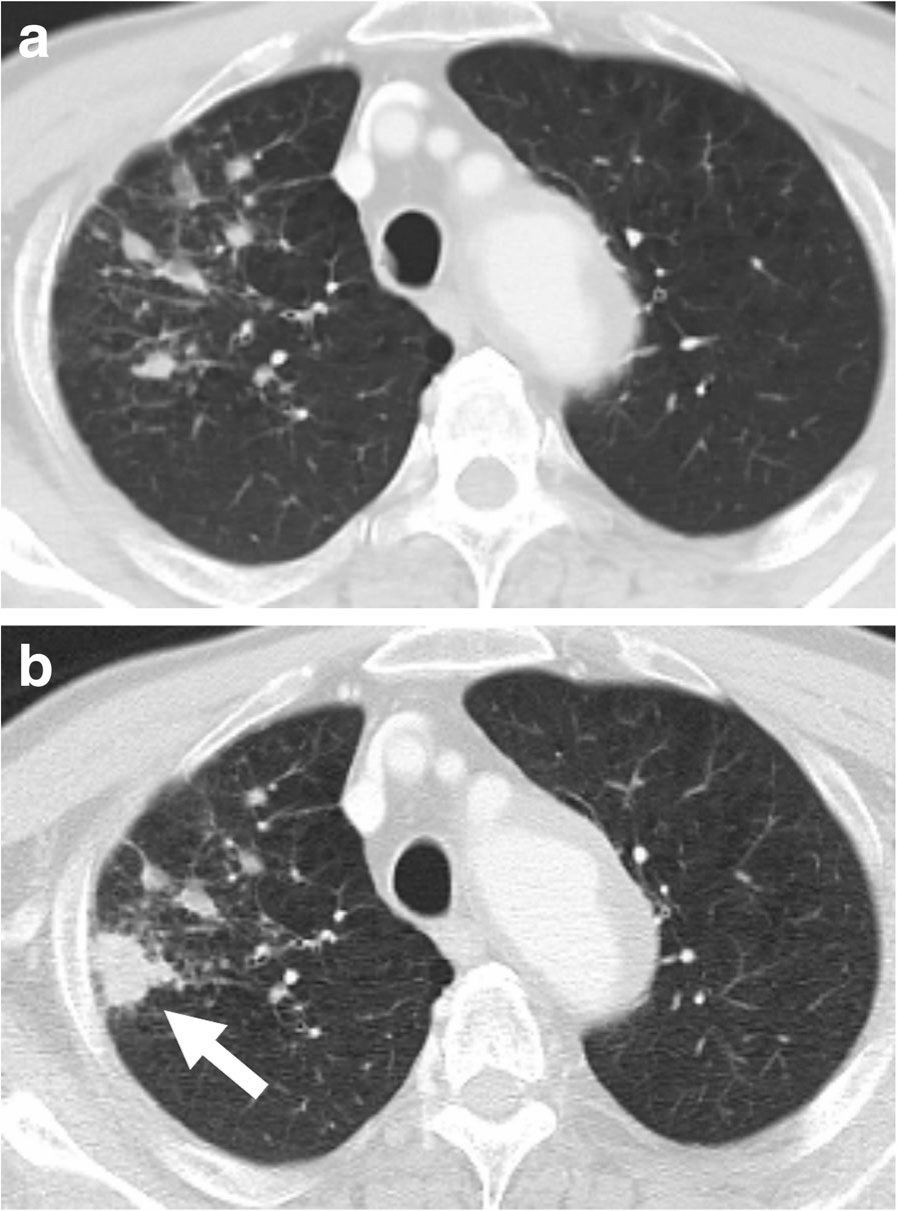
Chronological changes in the lungs for Case 2 as shown usingcontrast-enhanced computed tomography. a Multiple pulmonary metastases suspected in right lung. b Consolidation which appeared in a part of the pulmonary metastases. The arrow is pointing to the part of the tuberculosis infection
